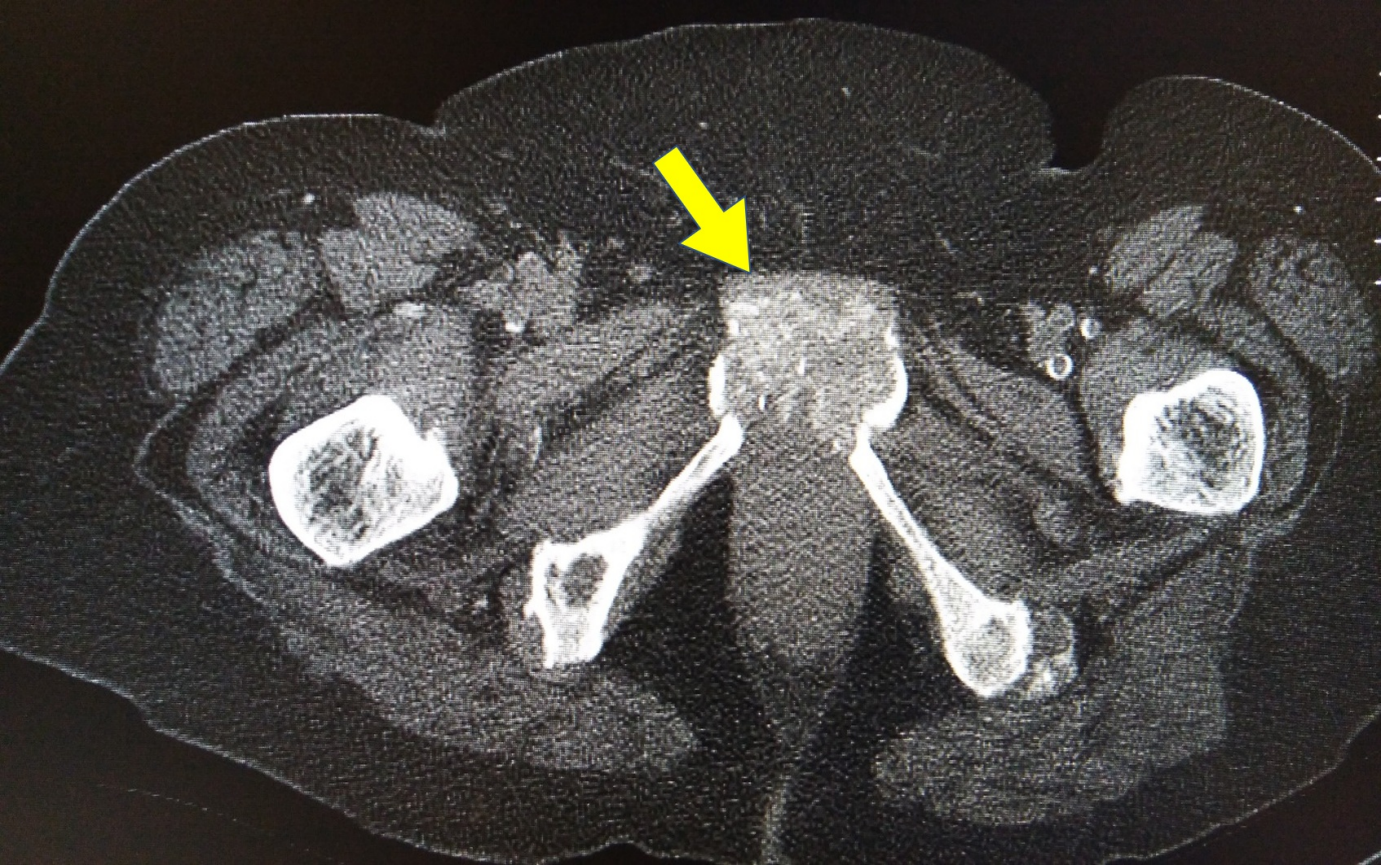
Computed tomography (CT) scan of the pelvis - coronal view Chronic symphysitis of pubic symphysis.
